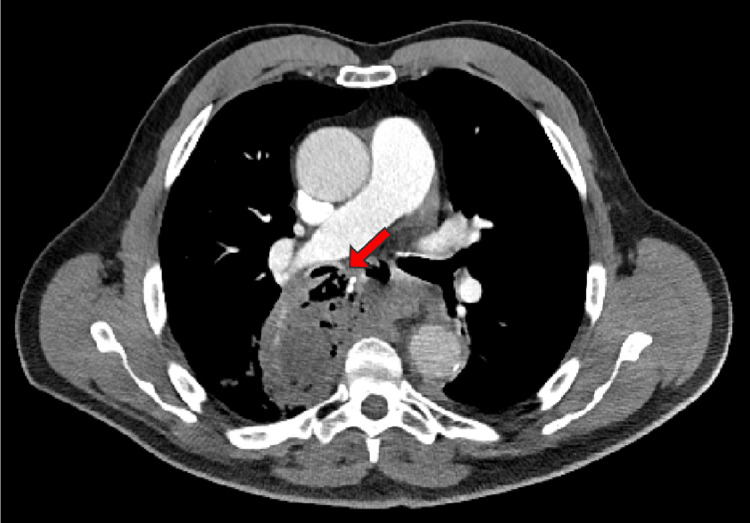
Axial view computed tomography (CT) of the thorax demonstrating the bronchoesophageal fistula (BEF) (indicated by the red arrow)
